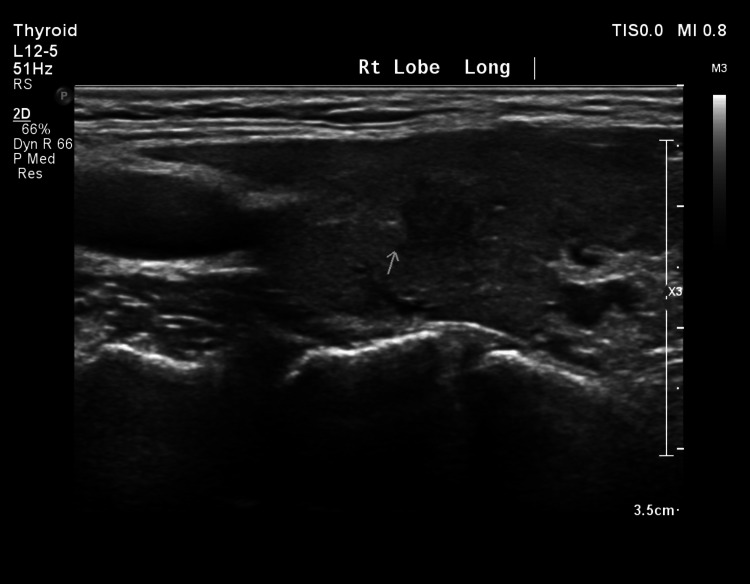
A long-axis ultrasonographic view of the right thyroid lobe demonstrates a central ill-defined hypoechoic area
